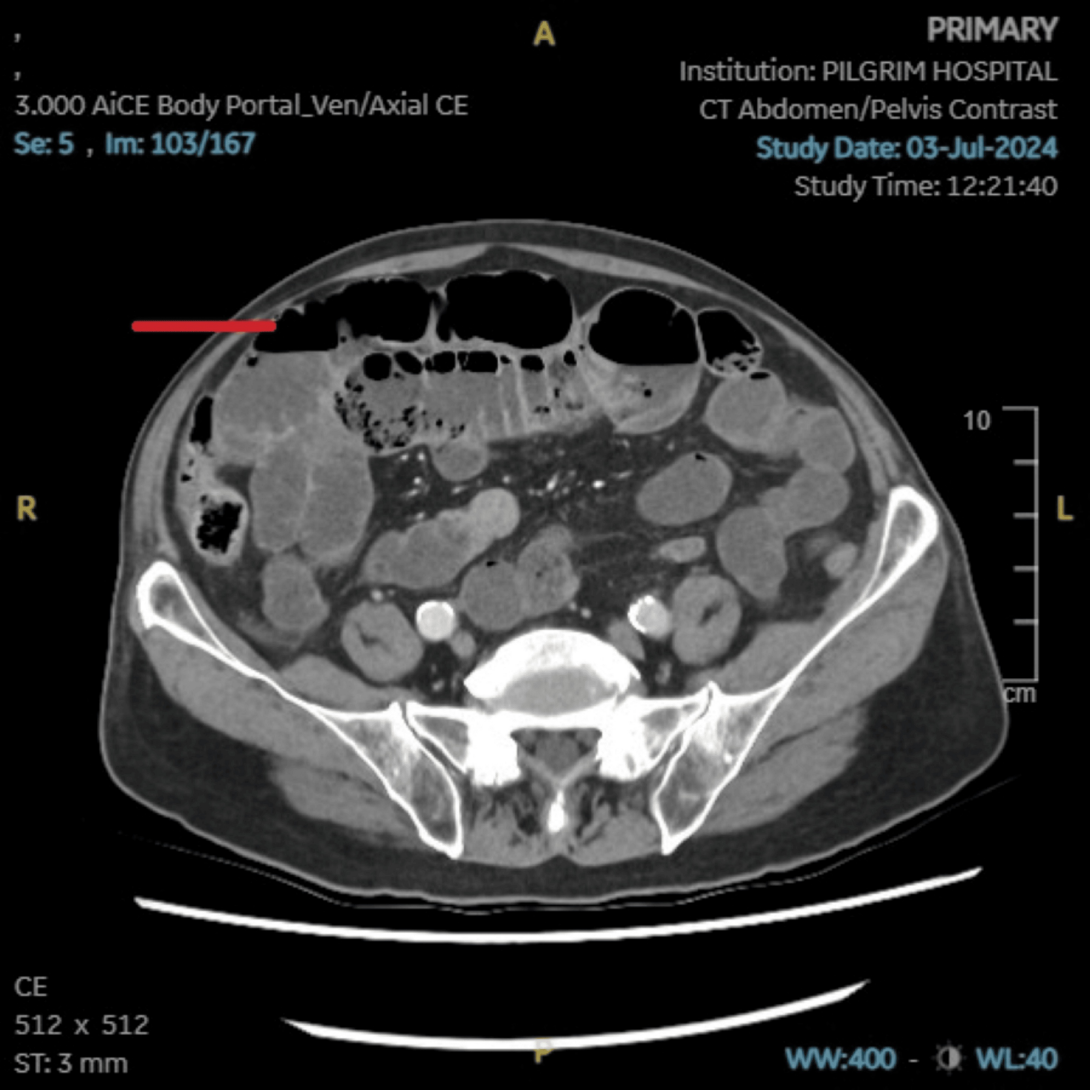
CT Abdomen pelvis with contrast. There is dilatation of the small bowel loops, with fluid distension of the stomach in the lower thoracic oesophagus. The distal small bowel loops are collapsed. The zone of transition appears to be in the mid abdomen (pointed red line).
